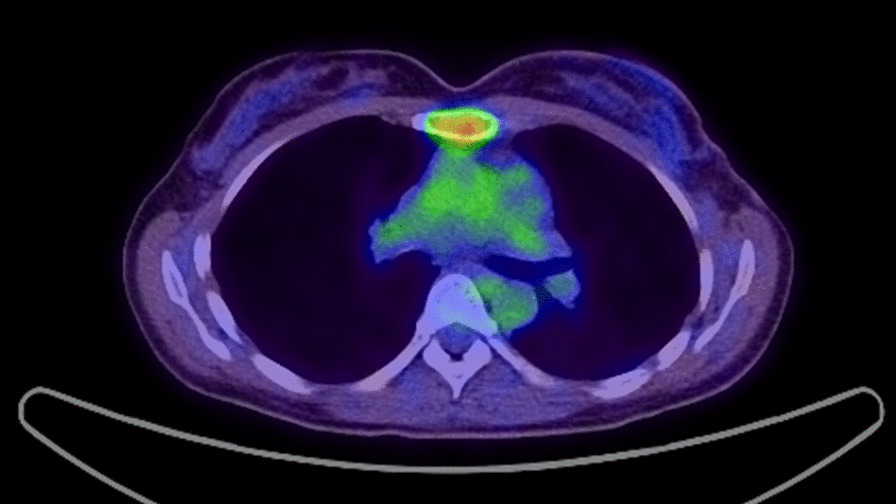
Positron emission tomography–CT revealed uptake of 18F-2-fluoro-2-deoxy-d-glucose in the sternum (maximum standardized uptake value of 4.5)
